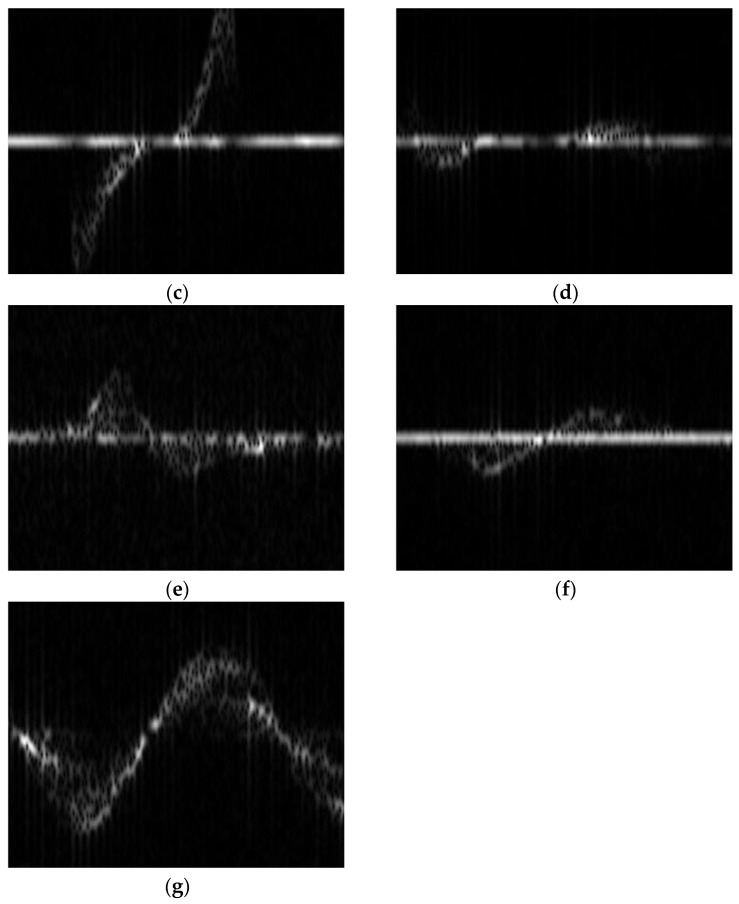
The micro-Doppler spectrograms of different actions, the images are as follows: (a) stationary; (b) head turning; (c) hand raised to ear; (d) hand raised to mouth; (e) head drooping forward; (f) head nodding; (g) leaning forward.
